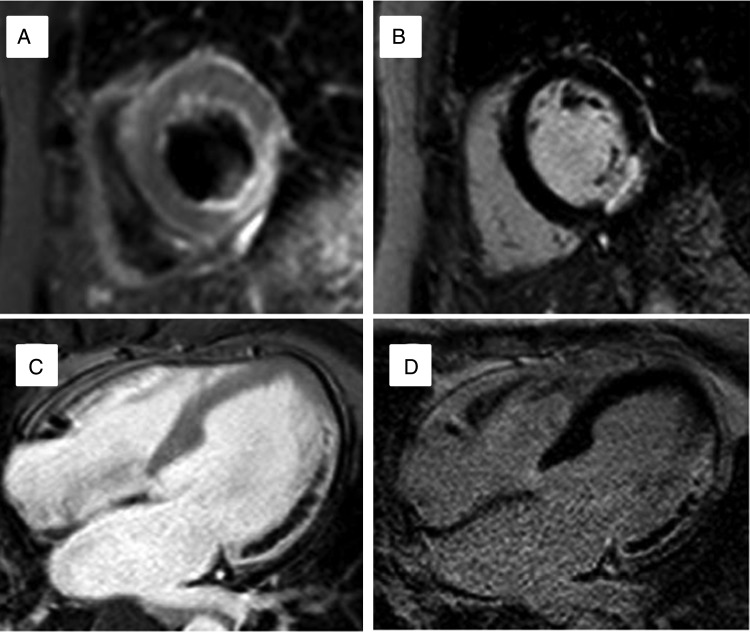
Acute myocardial infarction on cardiac magnetic resonance: Top row: (*A*) High signal on T2-weighted image demonstrating Infero-lateral oedema and ‘area at risk’. (*B*) Subendocardial infarction in the same patient on late gadolinium enhancement. Bottom row: (*C*) early gadolinium enhancement image in a second patient with extensive lateral wall hypoenhancement. (*D*) Late gadolinium enhancement confirms infarction and microvascular obstruction.
